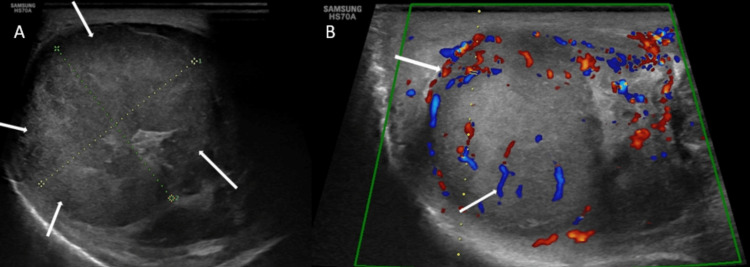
A: High-frequency ultrasound showing a heterogenous, predominantly hypoechoic mass in the right scrotal sac, replacing the right testis. B: On color Doppler, the mass was highly vascular.
